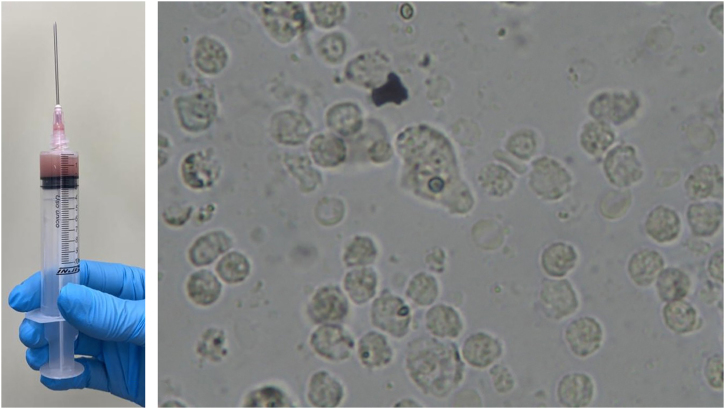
Direct examination of secretion collected by ultrasound-guided puncture (A) showing the parasite *Entamoeba histolytica* in the form of cysts and, in the center of the image (B), trophozoites.
